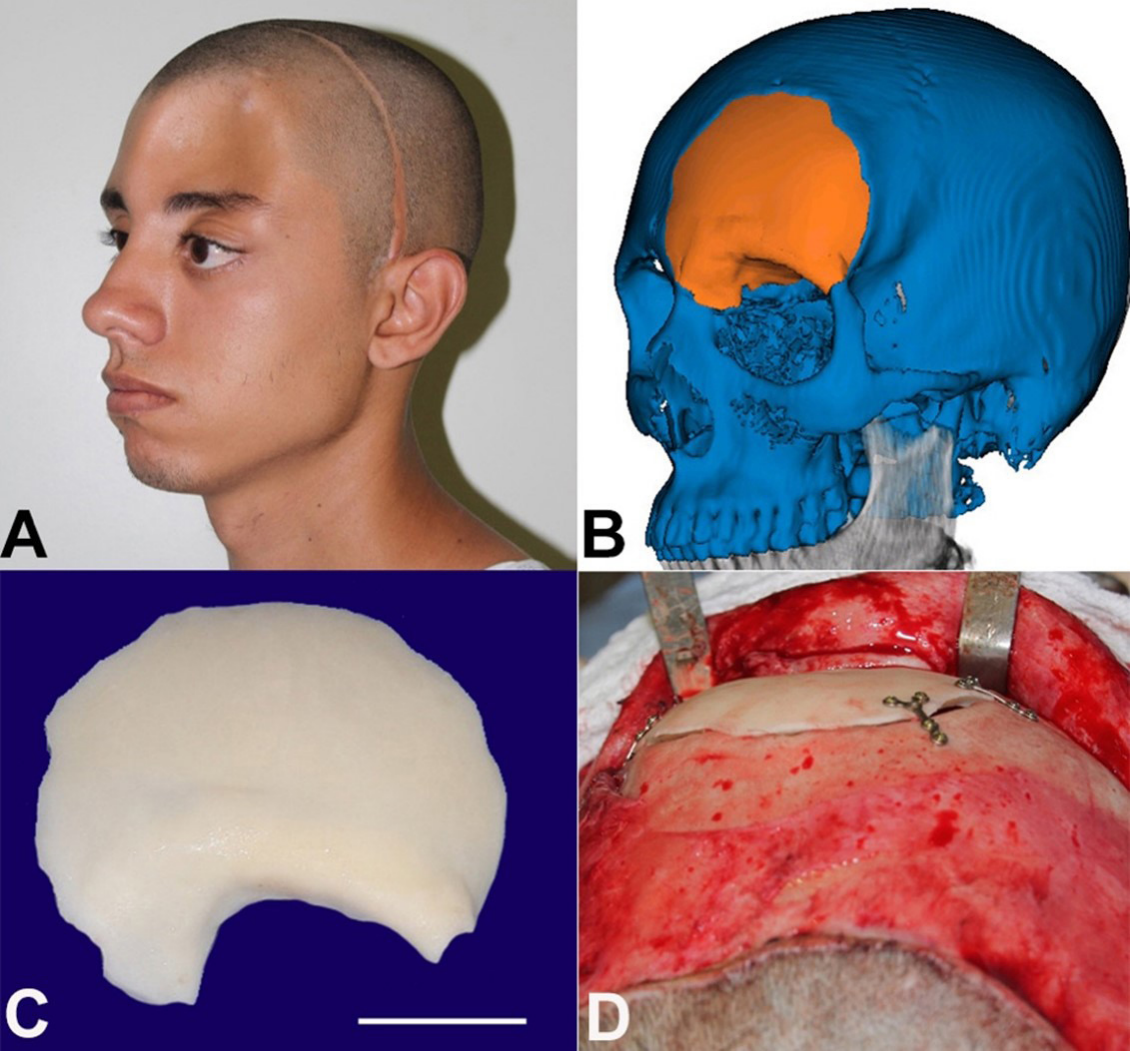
Planning and rehabilitation. A – Postoperative appearance after the first surgical intervention showing concavity with a noticeable aesthetic defect; B – Planning guided by CT; C – Polymethylmethacrylate implant (scale bar = 2,5 cm); D – Implant fixed with plates and screws from the fixation system1.5.
